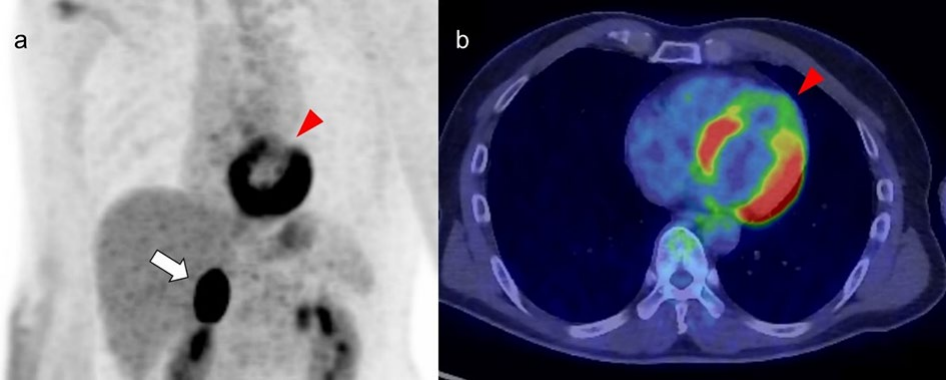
Catecholamine induced myocarditis. Focal FDG uptake is seen in the pheochromocytoma arisen at right adrenal grand (a white arrow). Decreased patty FDG uptake in the anterior wall to apex of left ventricle (a, b red arrowheads) indicated catecholamine induced myocarditis
